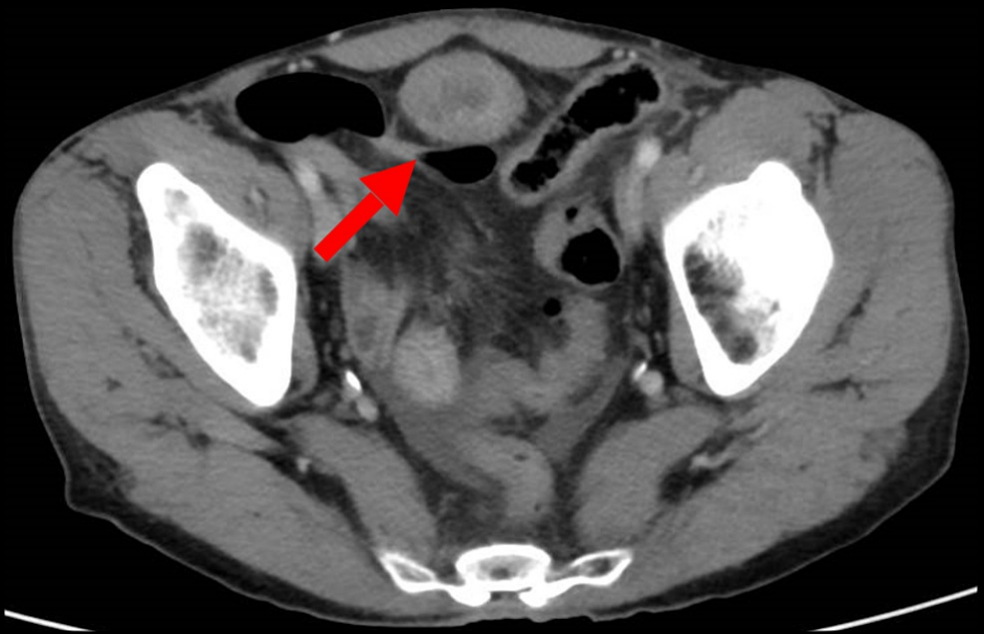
CT scan - transverse section (image 2) Arrow indicates the presence of urachal remnant CT: computed tomography
